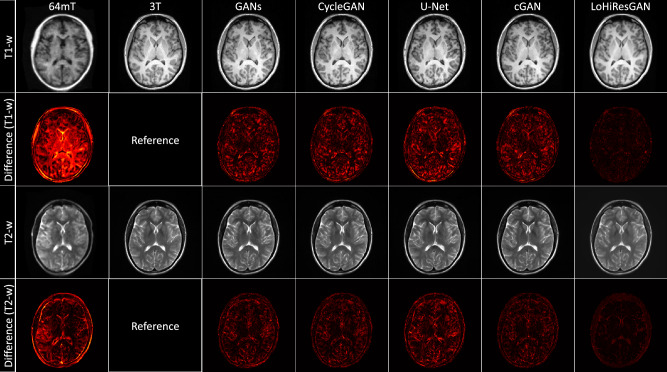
Comparison of T1-weighted and T2-weighted images (32-year-old male participant) and their absolute difference (plotted in the range of [0, 1]) with respect to 3T reference images across various state-of-the-art image-to-image translation techniques. From left to right: 64mT, 3T, GANs, CycleGAN, U-Net, cGAN, and LoHiResGAN.
